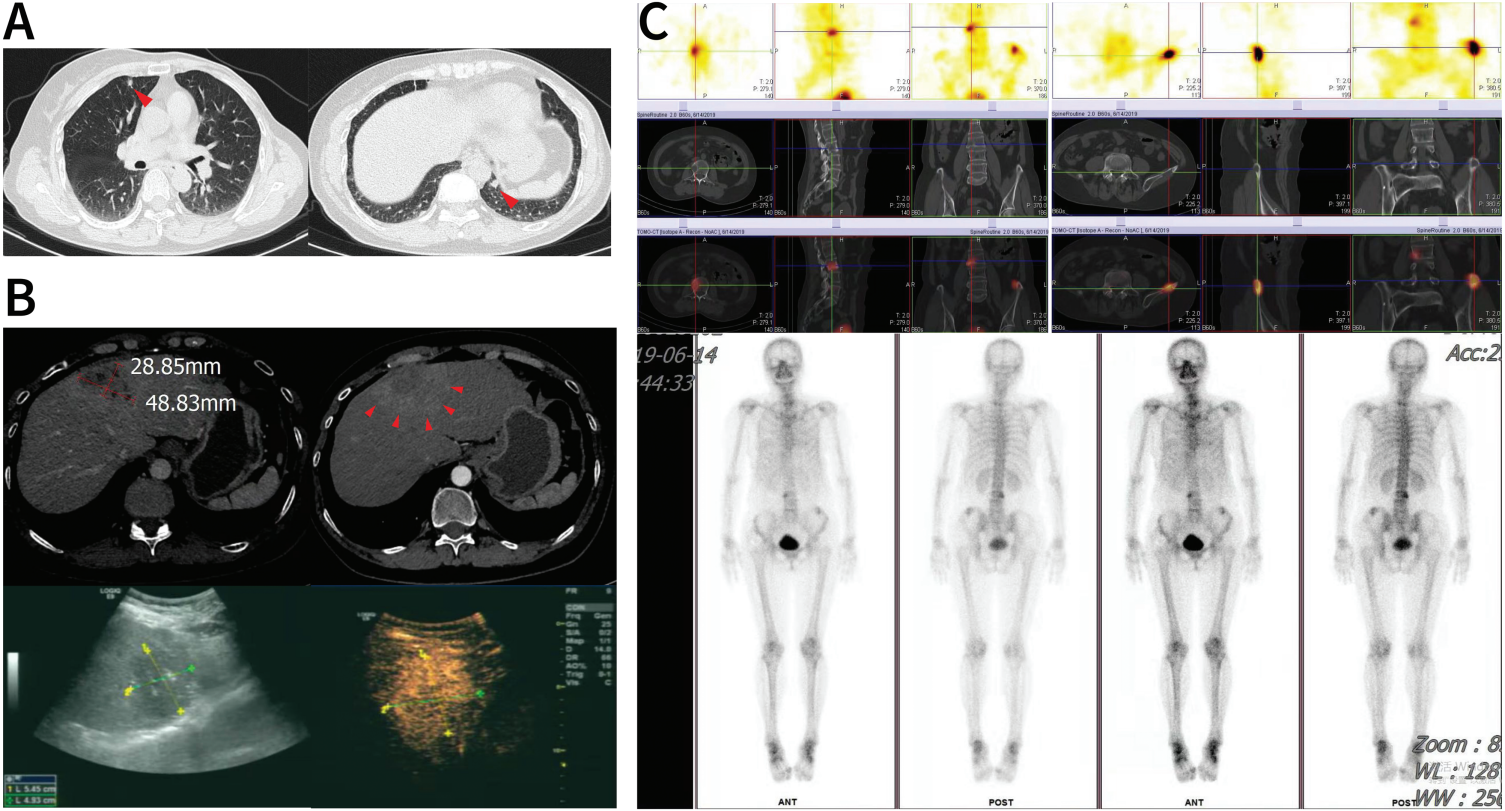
Representative images of lung, liver, and bone metastases at an early stage of systemic metastasis. A: Two lung metastases on CT scans (arrows); B: left liver metastases on CT scans (upper images, arrows) and contrast-enhanced ultrasound scans (lower images, arrows); C: bone metastases on whole-body bone scans (hot spots with increased radionuclide uptake can be observed in the first thoracic vertebra, the third lumbar vertebra, and left ilium).
